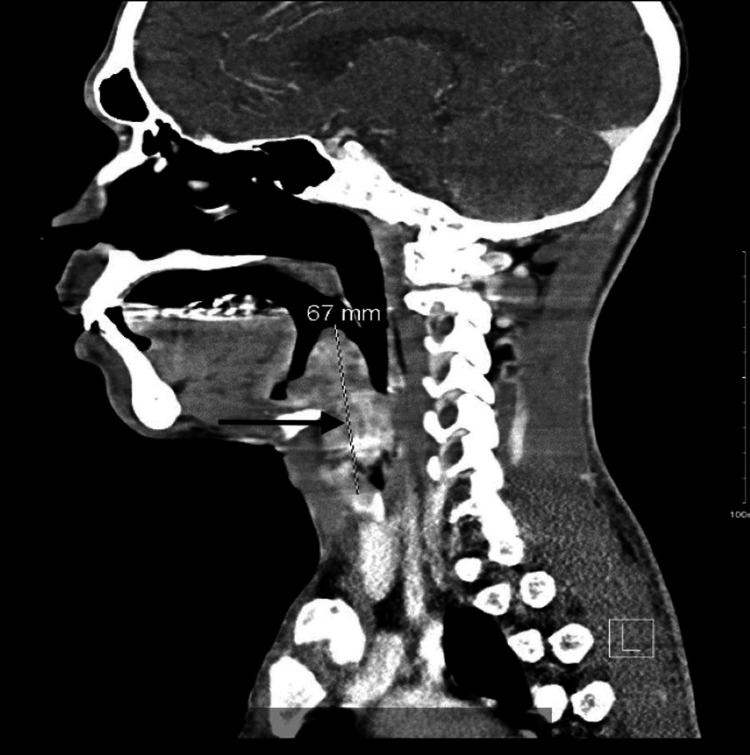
Contrast-enhanced computed tomography images (sagittal view) showing a lobulated mass arising from the epiglottis and extending to the oro- and hypopharynx. The mass lesion causes near-complete obstruction of the upper airway.
